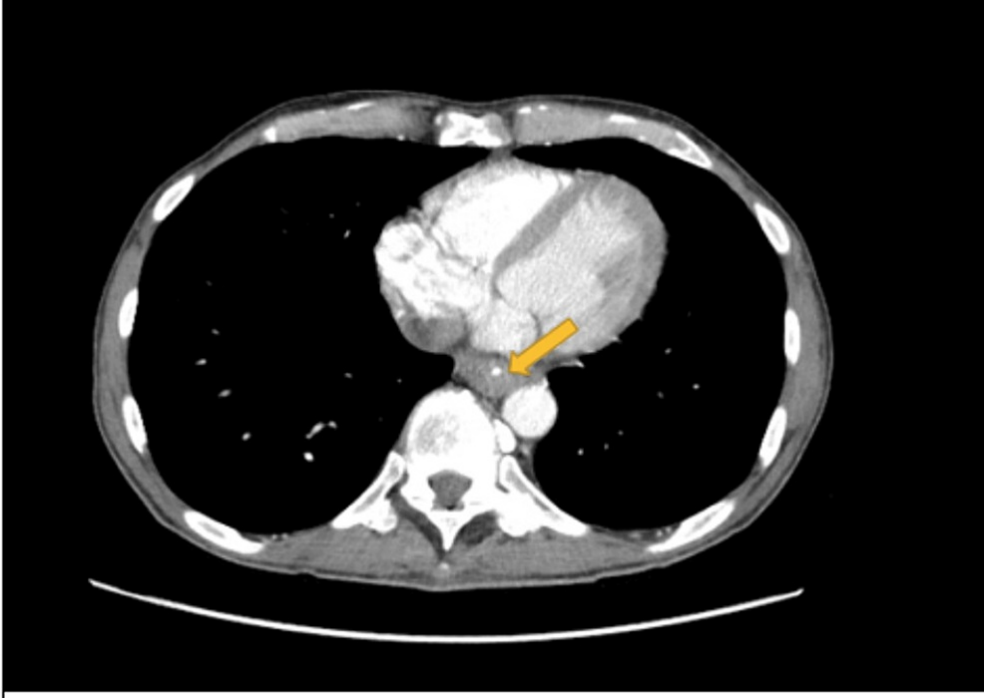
CT chest with contrast showing esophageal thickening (yellow arrow)
